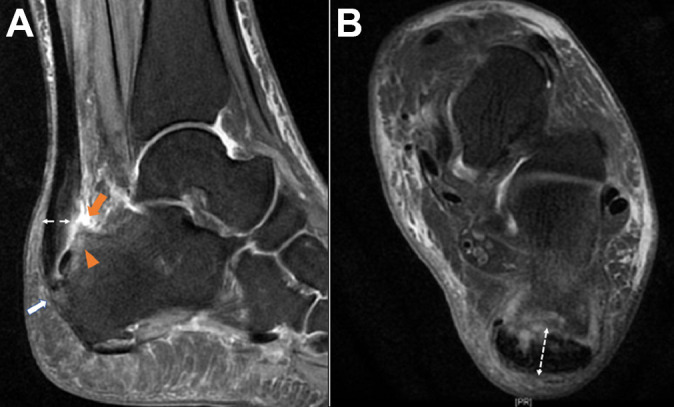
Signs of Haglund syndrome on (A) sagittal and (B) axial magnetic resonance imaging: retrocalcaneal bursitis (orange arrow), bone marrow edema (orange arrowhead), bony spur (white arrow), and Achilles tendon diameter (double-headed dashed arrow in both images). The intratendinous hyperintensity of the Achilles tendon in this patient was classified as Pomranz grade 1b.
